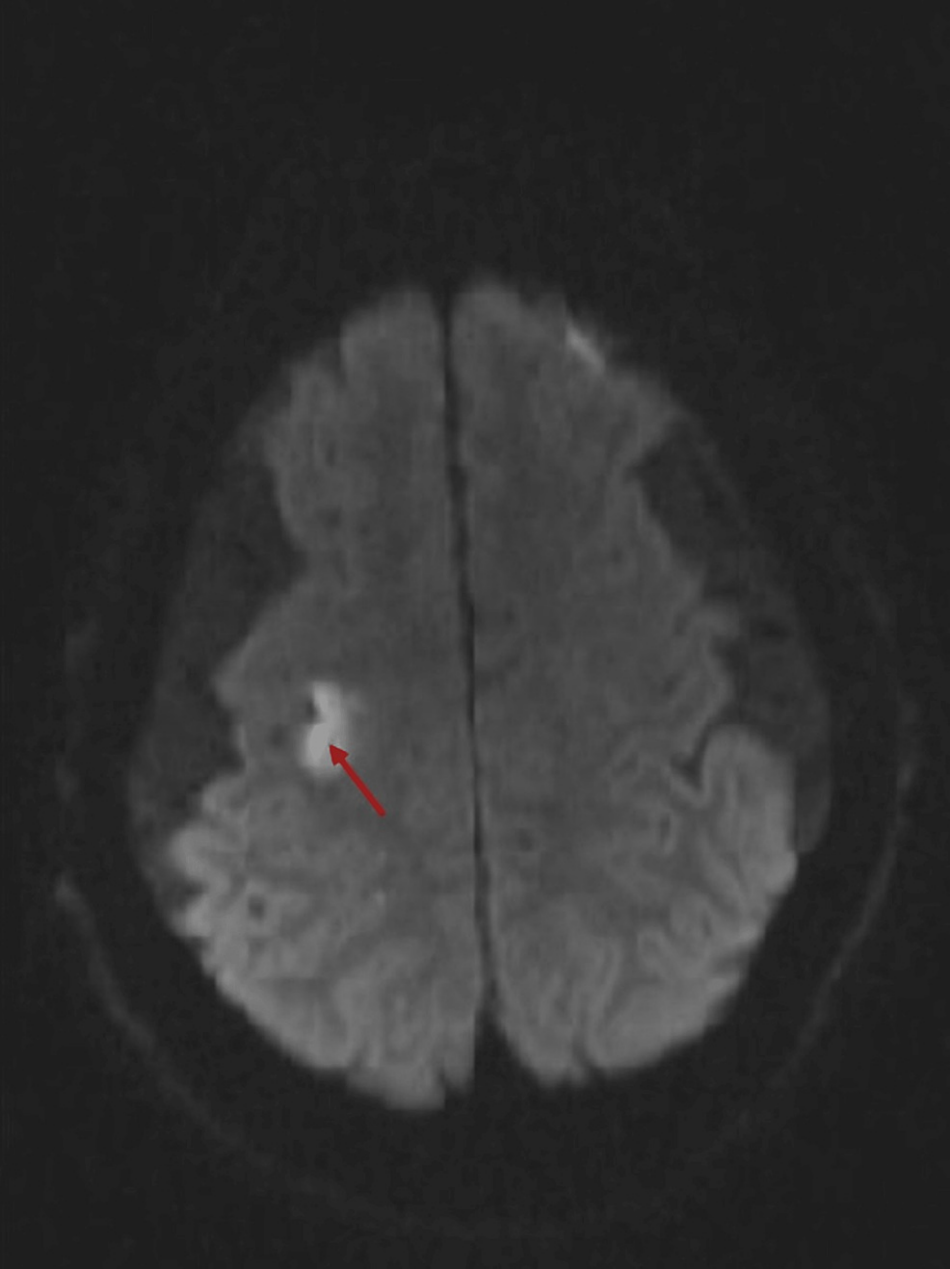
Brain MRI findings post-admission Diffusion-weighted MRI showing an acute infarction located in the “hand knob area” of the right precentral gyrus (red arrow). MRI, magnetic resonance imaging
